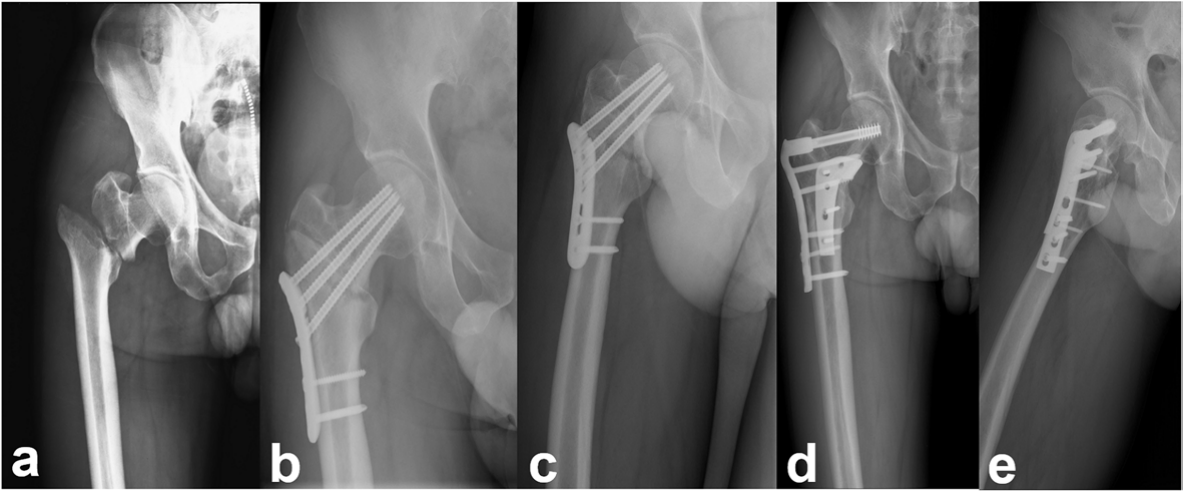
A 41-year-old man with right trochanteric fracture (AO/OTA A2) initially fixed by proximal femoral locking plate. a The X-ray of original fracture. b-c Nine months after surgery, X-rays showed fracture not yet healed. d-e The previous implant was replaced with a DCS and a MABP. d The X-ray showed the fracture healed three months after revision surgery
